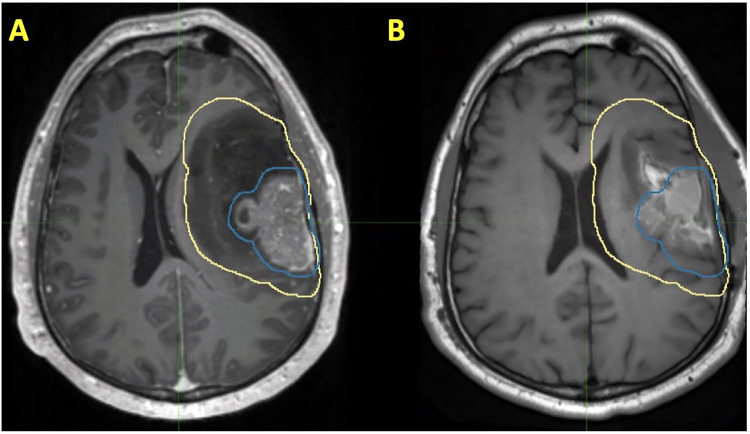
Patient with a history of glioblastoma, where the volume encircled in blue represents a preoperative SRS volume and the volume encircled in yellow represents a postoperative radiotherapy volume. Each of these volumes are shown on (A) preoperative T1 post contrast MRI and (B) T1 postoperative T1 post contrast MRI.
